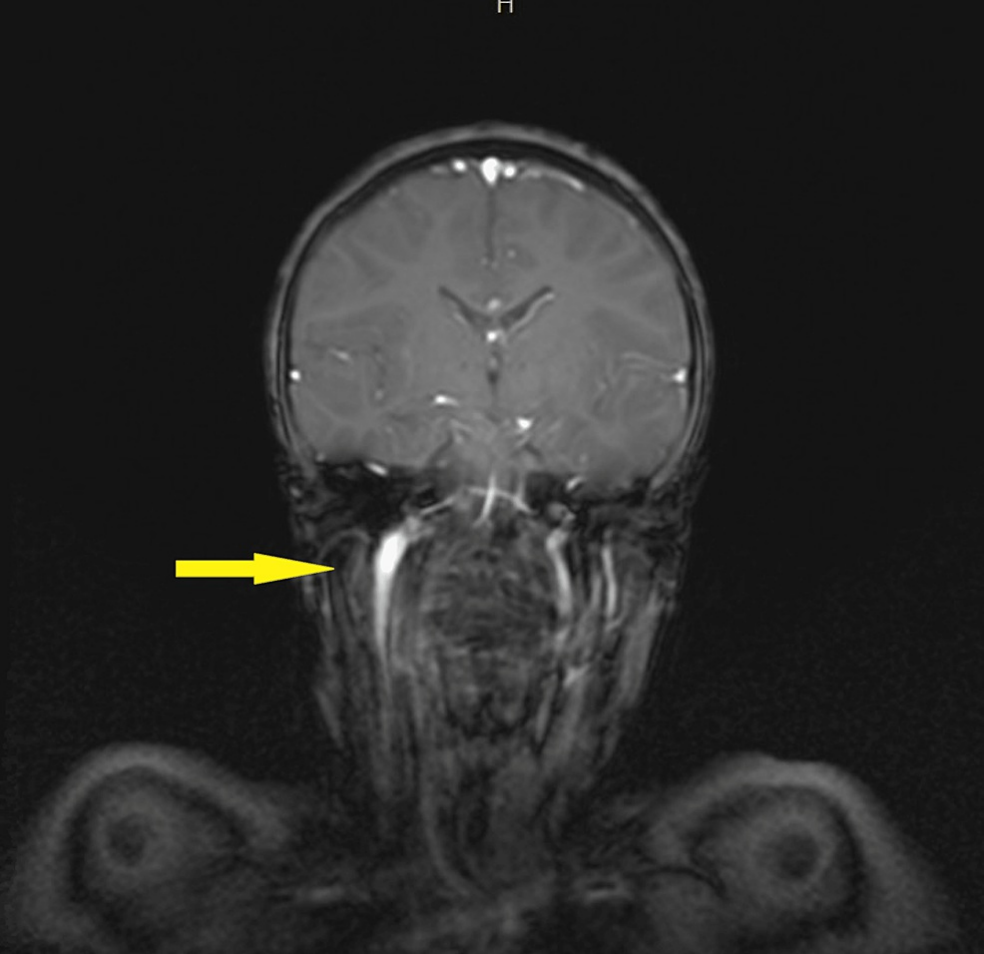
Computed tomography (CT) angiogram showed an impression of relative left peripheral vascularity compared to the right side
